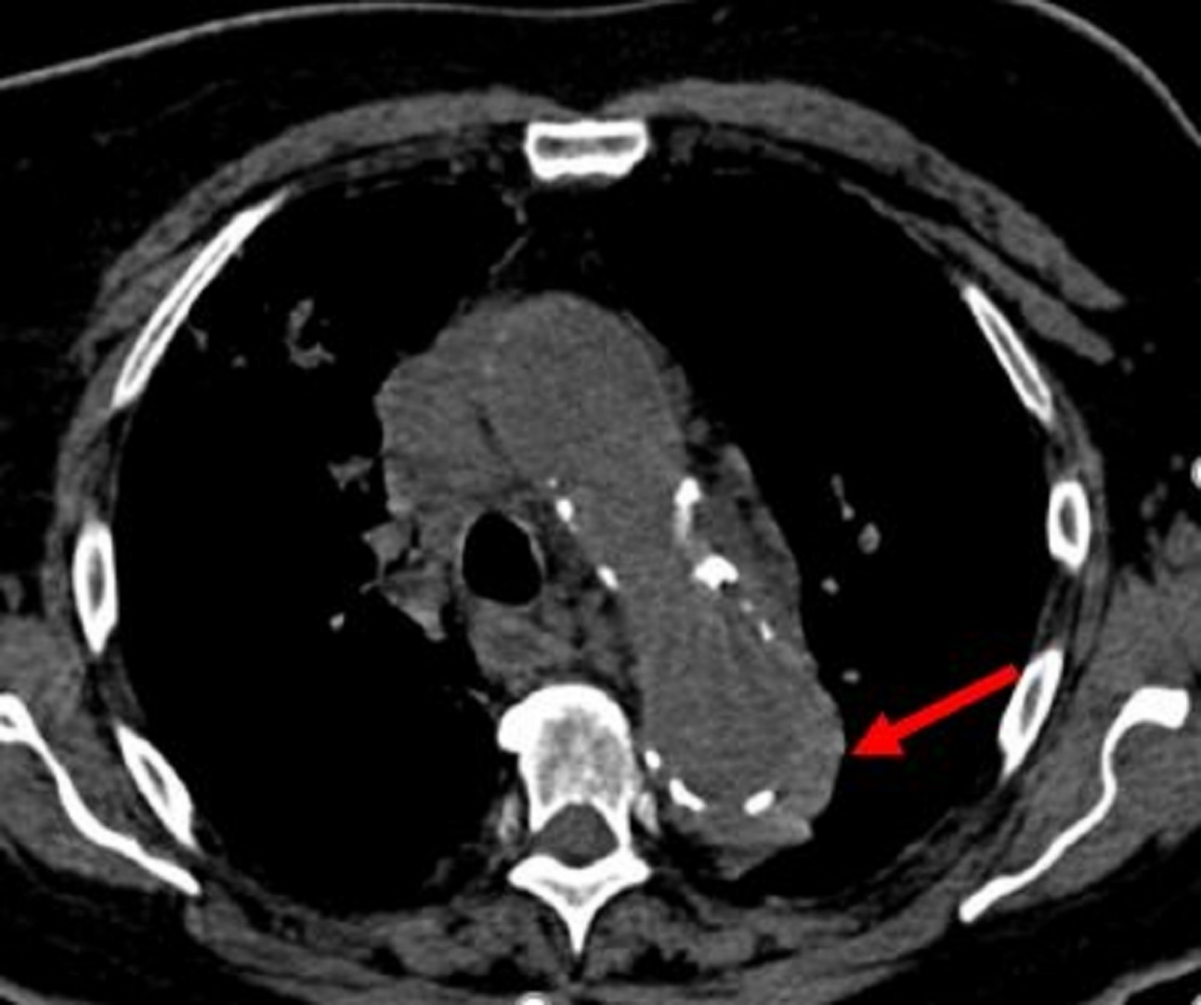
Non-contrast enhanced axial computed tomography at the level of the aortic arch and demonstrates a hyperdense ring around the aorta (red arrow) representing an intramural hematoma.
